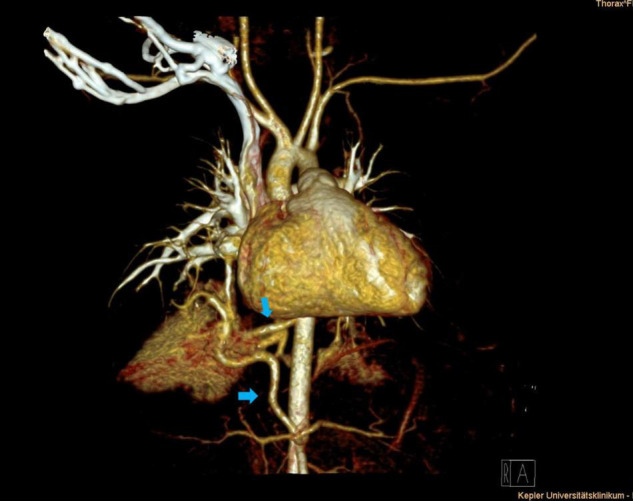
Thoracic contrast-enhanced computed tomography scan (CECT) depicting right lower lobe hybrid lesion. Supra- and infra-diaphragmatic arteries originate from the descending aorta and the celiac trunk (blue arrows).
